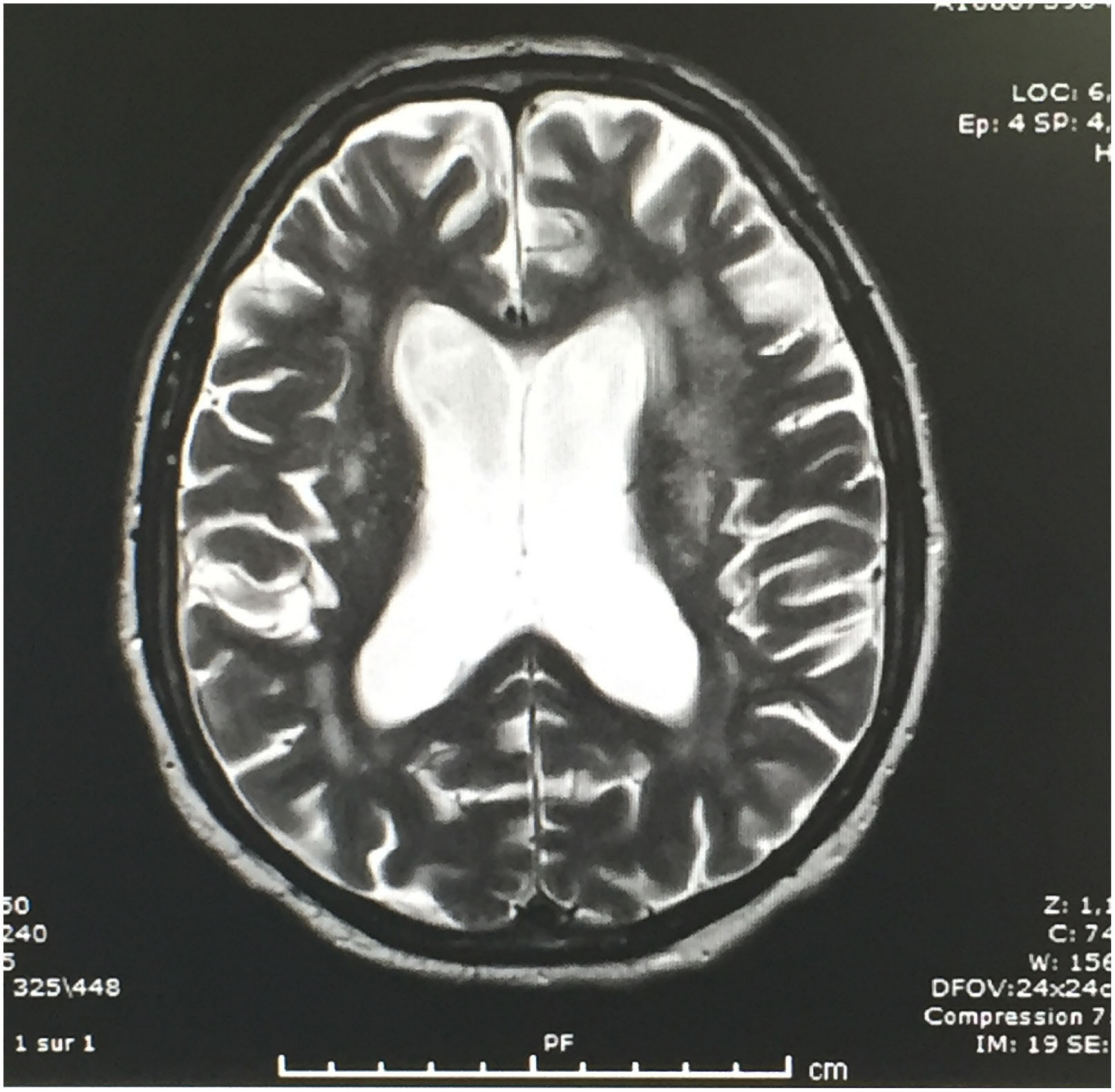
T2-weighted brain MRI: Note periventricular hyperintensities in relation with CSF resorption.
